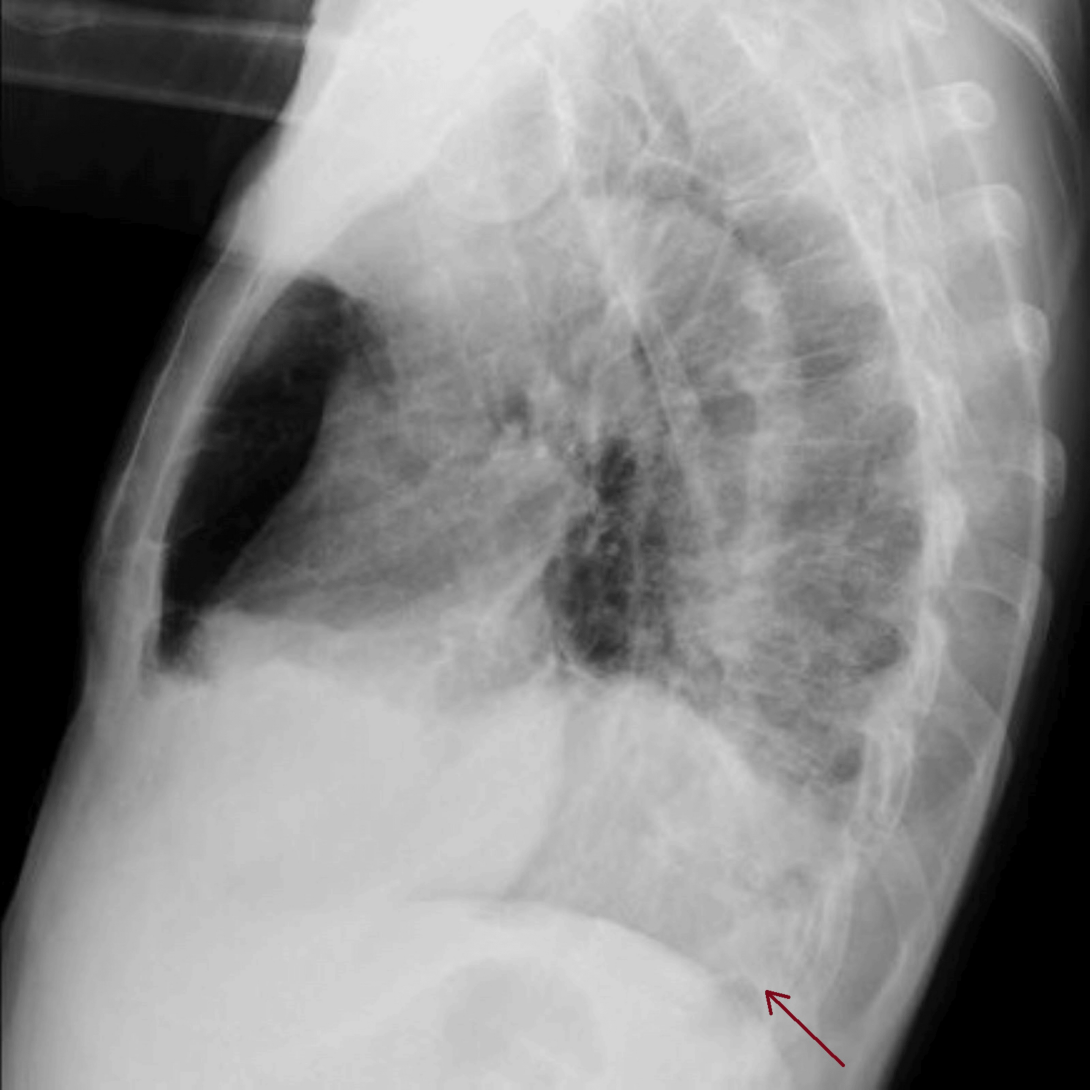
Chest X-ray performed on the day of admission suggests a right pleural effusion (arrow), lateral view.
